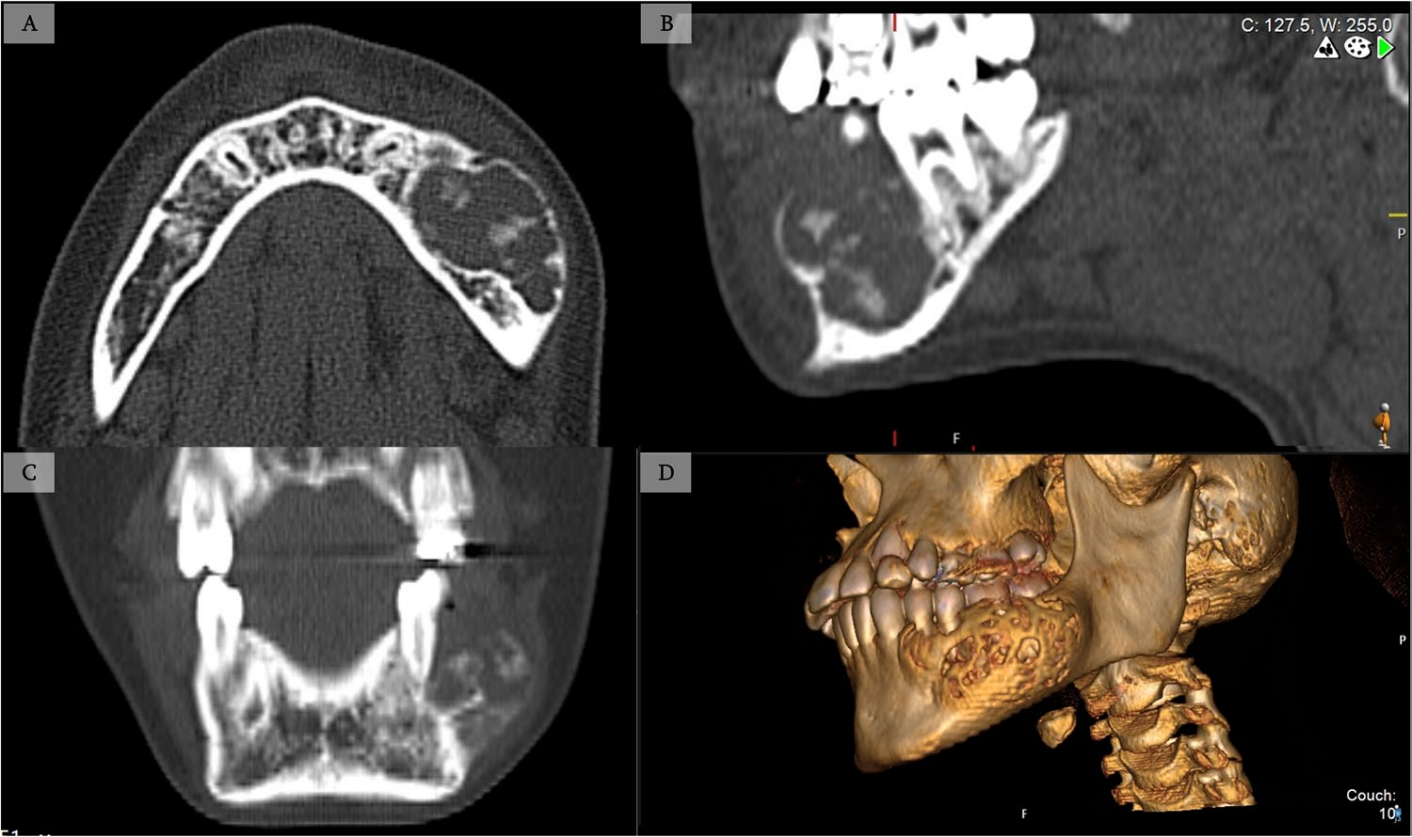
CT imaging following the treatment course of the patient described in Fig. 11. (A) Axial (B) Sagittal (C) Coronal and (D) 3D reconstruction, demonstrating the aggressive lesion post pharmacological therapy with intralesional injection and nasal calcitonin as detailed previously
